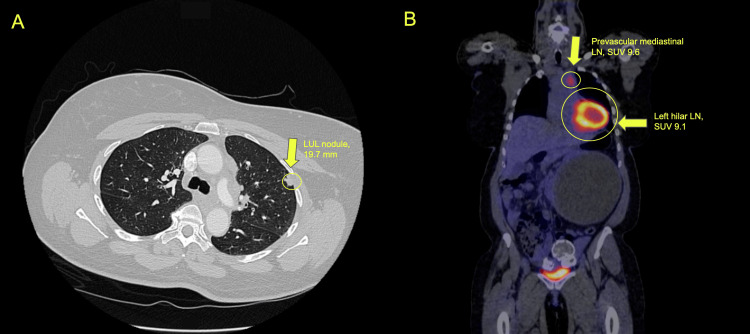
Chest computed tomography (CT) and positron emission tomography (PET)/CT demonstrating the primary left upper-lobe pulmonary lesion and associated FDG-avid nodal disease preceding nondiagnostic sampling. (A) Axial lung-window CT demonstrating a lobulated solid nodule in the left upper lobe measuring approximately 19.7 mm. (B) Coronal fused PET/CT demonstrating intensely FDG-avid left hilar lymphadenopathy with SUV 9.1 and prevascular mediastinal lymphadenopathy with SUV 9.6. The primary left upper-lobe lesion demonstrated comparatively mild FDG uptake with SUV 2.0. Annotations identify the primary pulmonary lesion and FDG-avid nodal regions. CT: computed tomography; PET: positron emission tomography.
